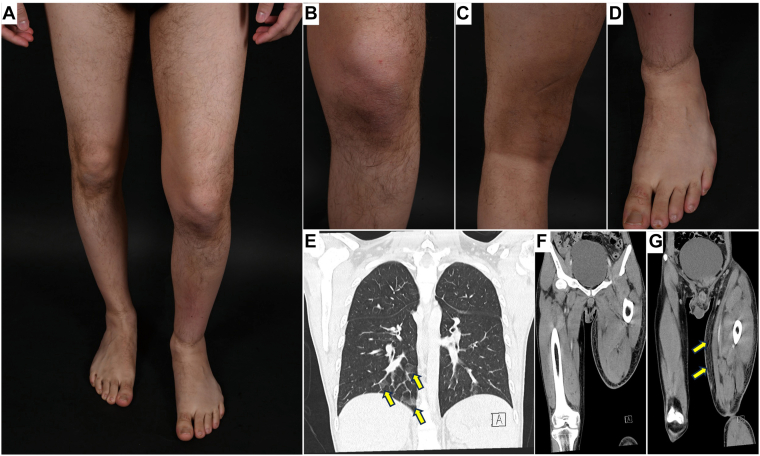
Sclerodermatous skin changes of the left leg and computed tomography (CT) scan. (A) Overview image and (B–D) close-ups demonstrating scleroderma-like skin changes of the left leg. (E–G) CT scan 2 days after hospitalization. (E) Lung CT shows ground-glass opacities in the lower lobes bilaterally. (F, G) CT of the lower extremities demonstrates diffuse edematous infiltration of the subcutaneous fat in the left thigh.
